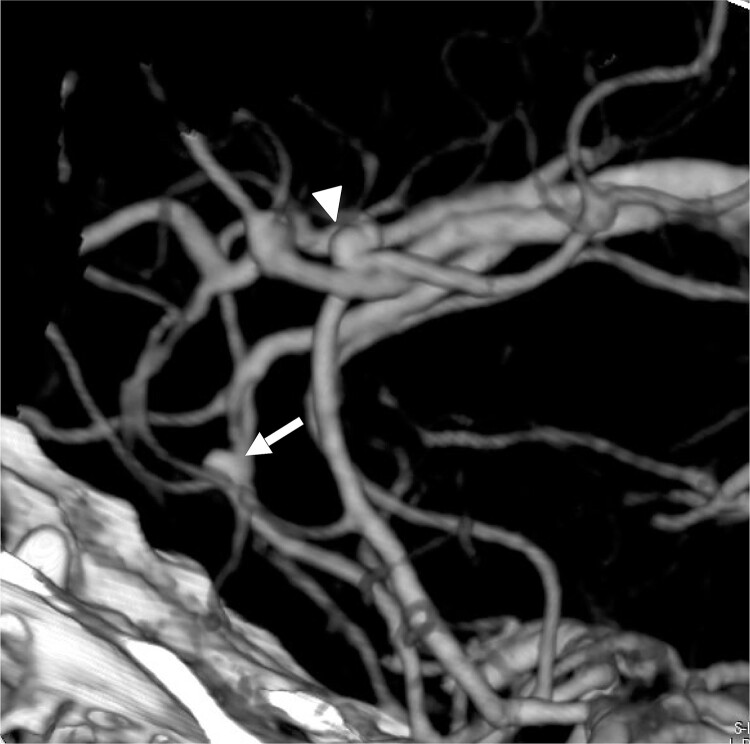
Lateral view (left to right) of three dimensional CT angiography taken at our clinic, showing cerebral aneurysm in the ACA of right A2A3 (white arrow) and left A3A4 (white arrow head).
